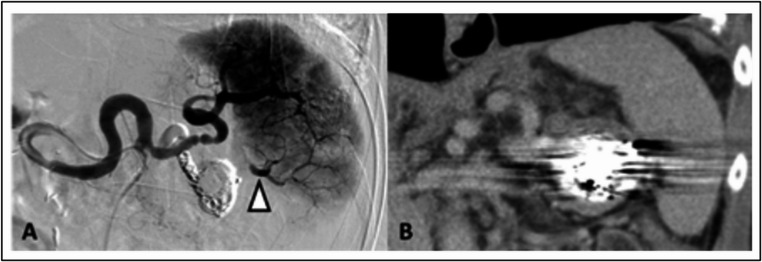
Technical success after coil embolization of the SAA. (A) Digital subtraction angiography confirming complete exclusion of the sac and the aneurysm-bearing main inferior branch artery with preserved perfusion of the spleen; note retrograde perfusion of the inferior lobar branches via anastomosing intrasplenic segmental branches from the main superior branch artery (white arrowhead). (B) Coronal contrast enhanced computed tomography image in the venous phase four days after SSA embolization confirming preserved perfusion of the entire spleen
